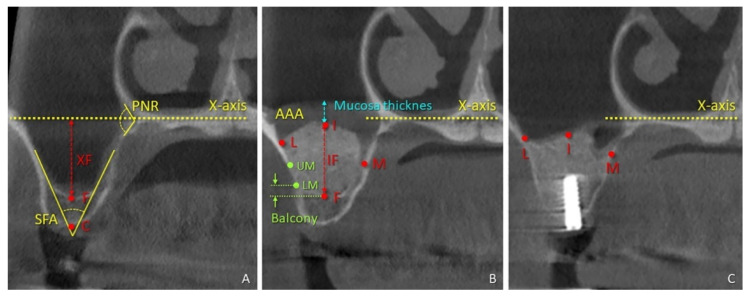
References for the measurements performed on CBCTs (A) before surgery, (B) after 1 week (B), and (C) 9 months. *X*-axis, plane placed at the base of the nasal floor; PNR, palate-nasal recess; F, sinus floor; C, top of the bone crest; XF, distance between *X*-axis and F; SFA, sinus floor angle; UM, upper margin of the antrostomy; LM, lower margin of the antrostomy; L, I, M, the uppermost extension of the hard tissue within the elevated space at the lateral, intermediate and medial aspects, respectively; AAA, alveolar-antral artery. Light green arrows, height of the balcony; light blue arrow, mucosa thickness.
